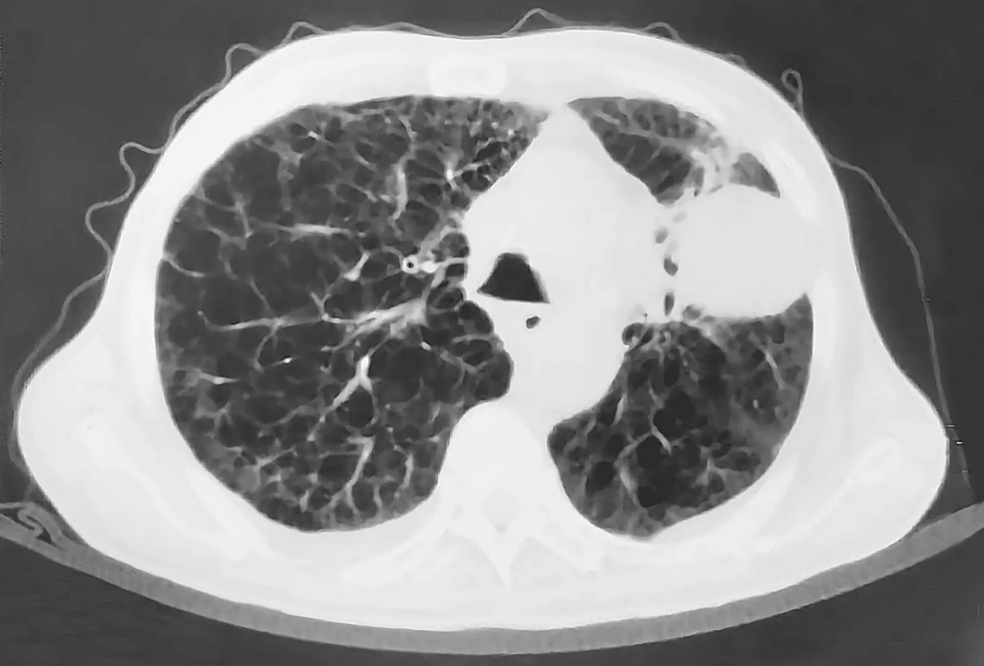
HRCT scan of Patient #2 (2.9× magnification). HRCT: high-resolution computerized tomography
